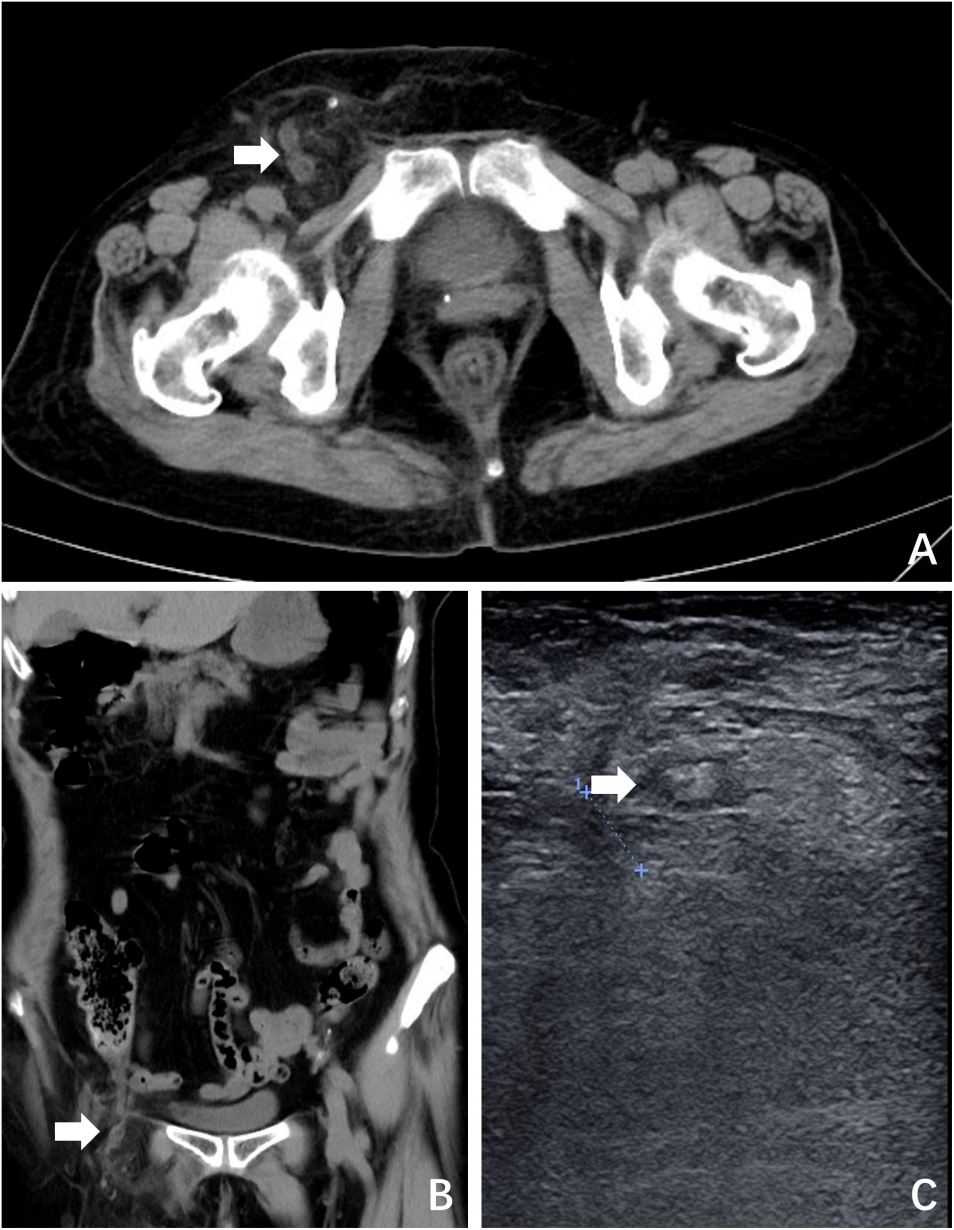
Preoperative imaging. (A) CT revealed a right femoral hernia defect inferior to the inguinal ligament and lateral to the pubic tubercle, containing the herniated appendix (white arrow); (B) coronal plane view of CT; (C) ultrasonography identified an incarcerated right femoral hernia containing the appendix (white arrow).
